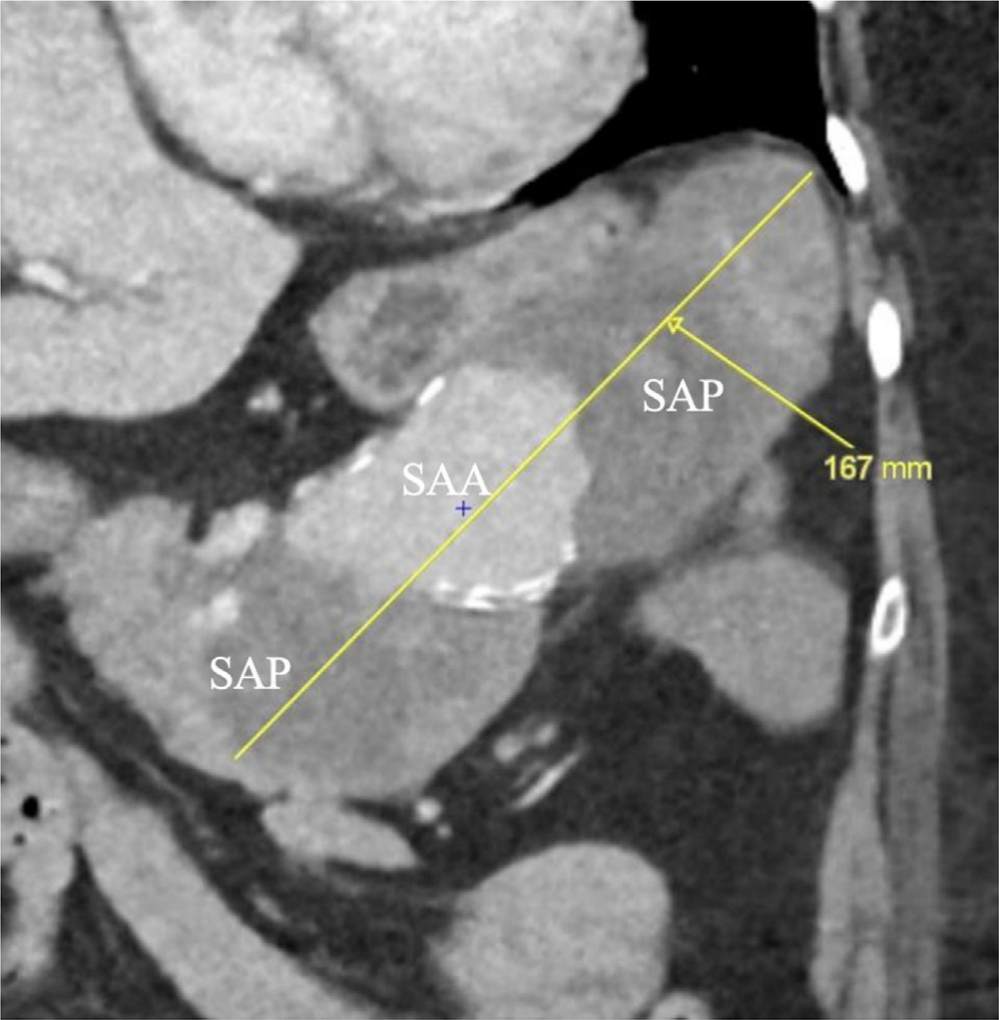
Contrast-enhanced CT, oblique MPR along the long axis of the lesion, demonstrating a giant pseudoaneurysm (SAP) surrounding the saccular splenic artery aneurysm (SAA), measuring ≈16.8 cm in maximal length.
